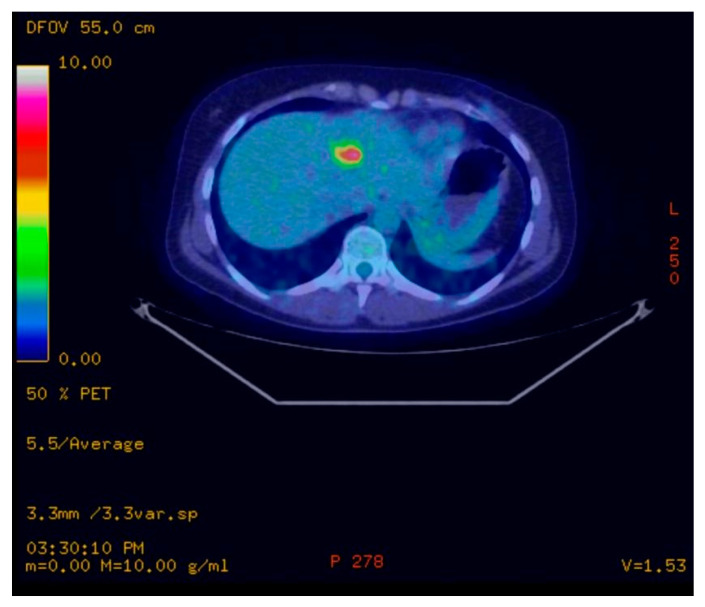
PET/CT scans performed in 2017 showing a single liver lesion of 22.3 mm in the 2nd segment with intense metabolic activity (SUV = 6.4) described as a single liver metastasis.
